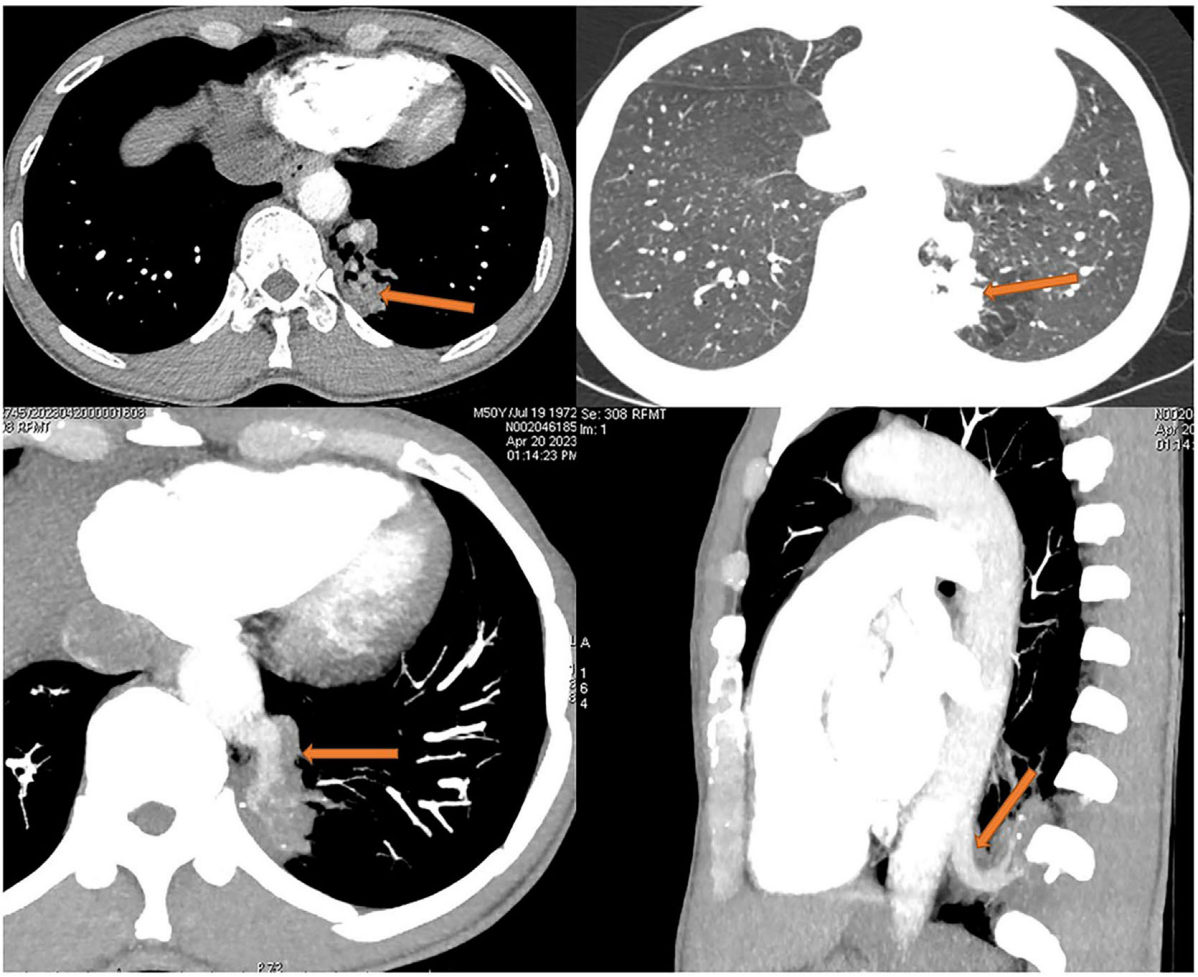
The thick branches of the thoracic aorta with a diameter of 15 mm entered the lesion. Chest enhanced CT combined with three‐dimensional vascular reconstruction shows that the posterior basal segment of the left lower lobe has patchy high‐density and unclear boundaries, and the descending thoracic aorta has a large branch with a diameter of 15 mm into the lesion.
